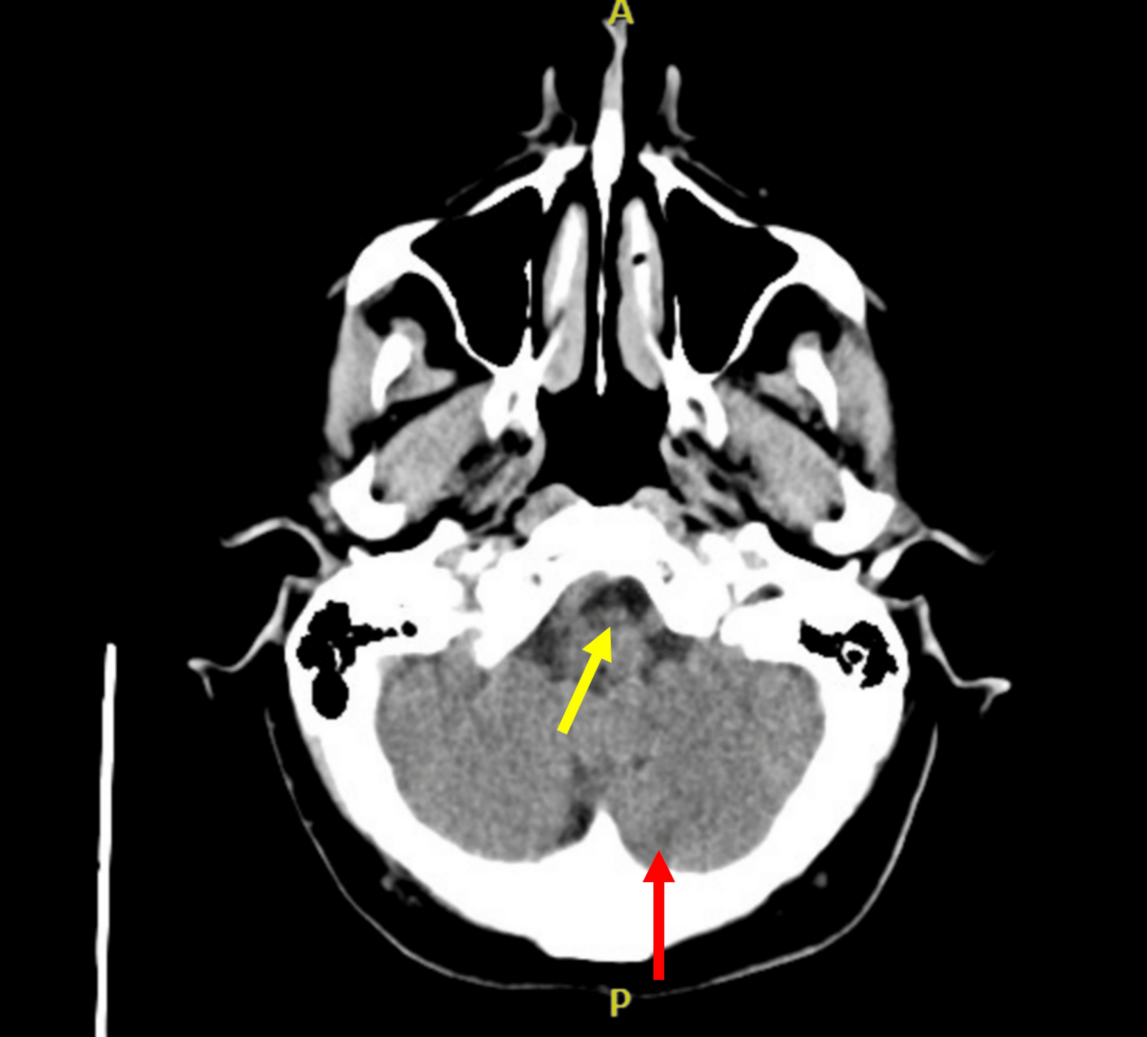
CTA head taken on day of admission showing focal severe stenosis of the left A2 segment, moderate stenosis of the left distal P1 segment, and a severe stenosis of the proximal left P3 segment (red arrow), and a 2 mm basilar tip aneurysm (yellow arrow) CTA: computerized tomography angiopathy
